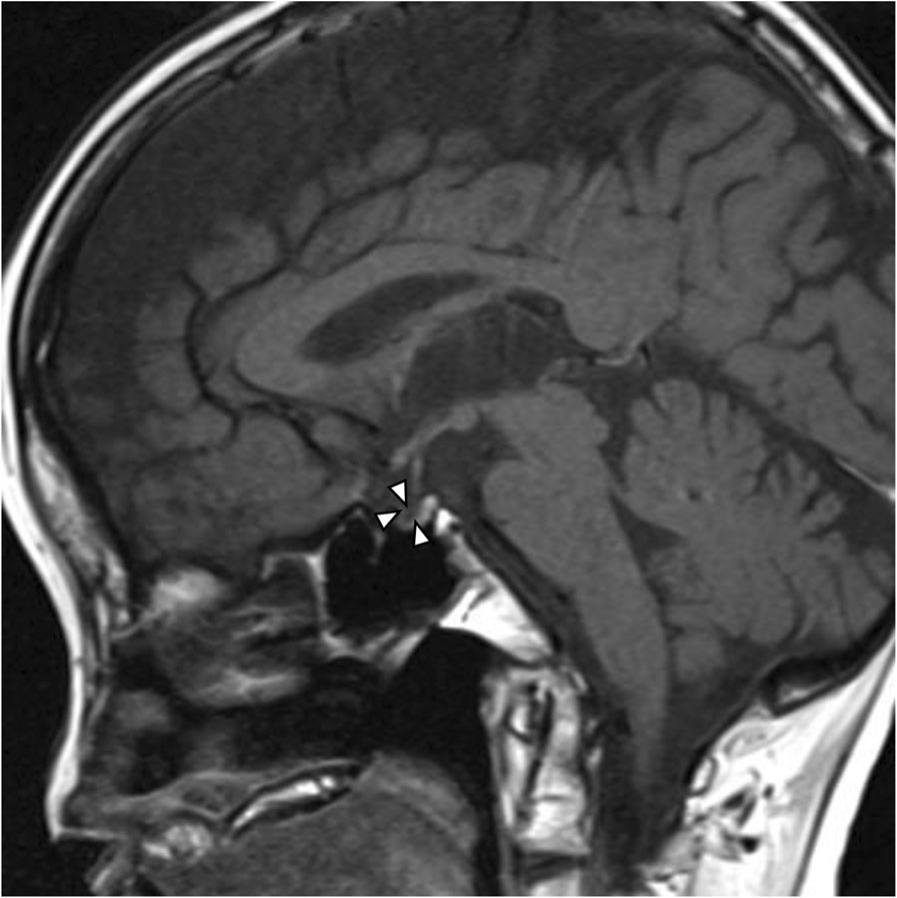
Hypothalamic-pituitary MRI image. A sagittal T1-weighted image is shown, confirming an empty sella turcica. No abnormality in the hypothalamus-pituitary stalk is evident, and a high-intensity signal is present in the posterior lobe. The anterior lobe is atrophic. Arrowheads indicate an empty sella turcica
